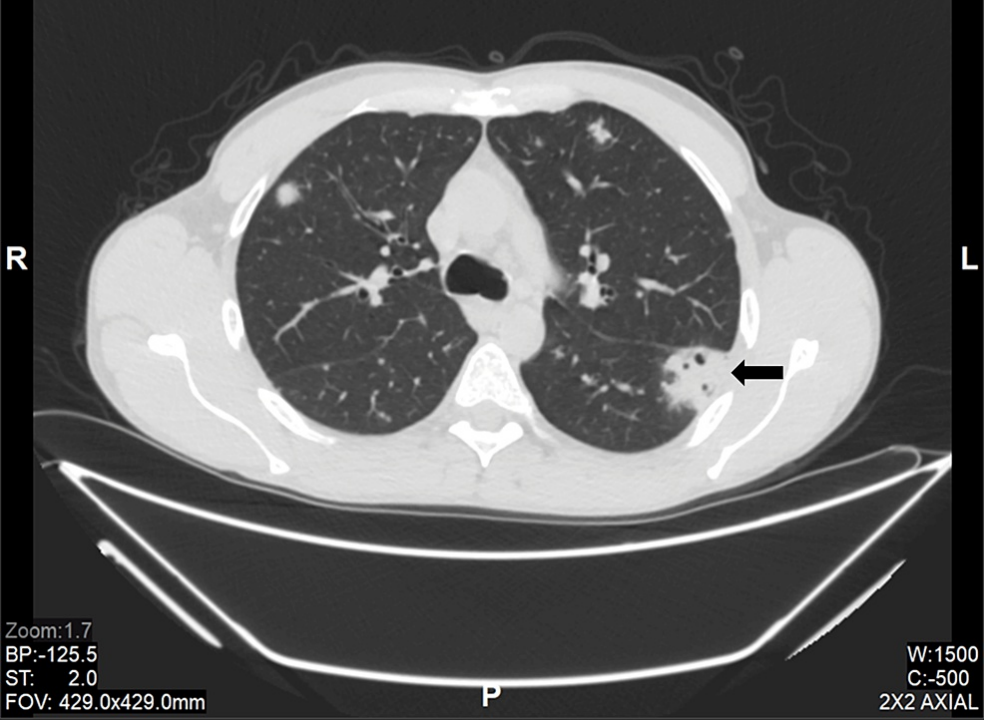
Non-contrast CT of the thorax, lung window Pulmonary nodules with fluffy margins are located in the periphery, and a cavitating lesion is also seen (arrow).
